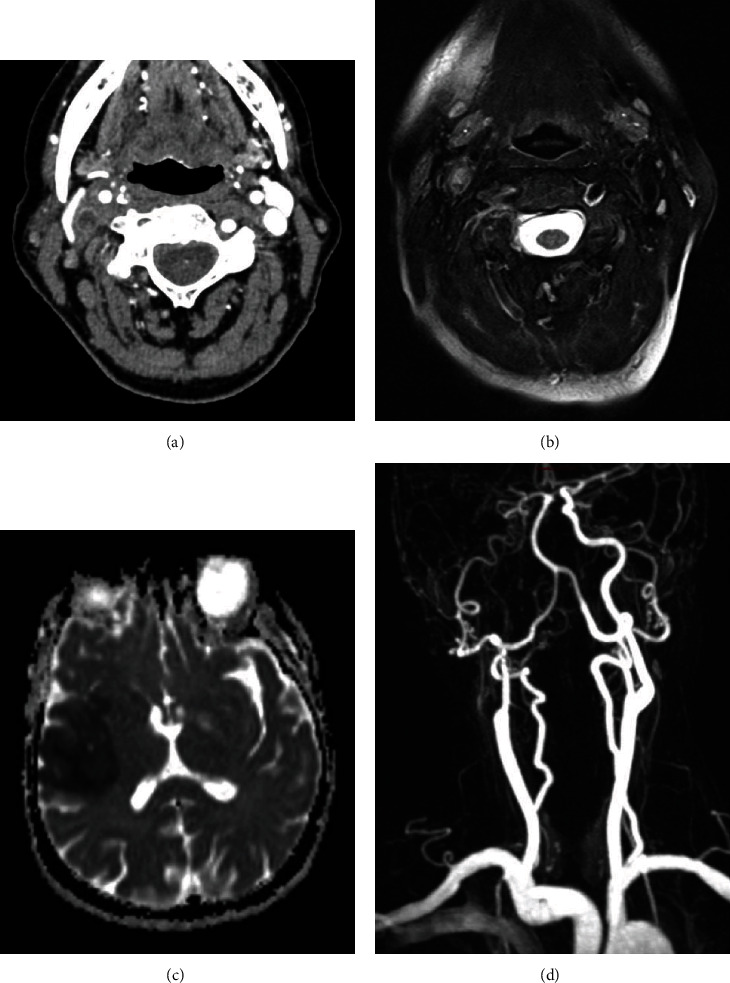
Axial postcontrast CT image (a) demonstrated the presence of vascular occlusion of the right internal carotid artery, immediately above the bifurcation, than confirmed by T2-weighted turbo spin echo (TSE) axial sequence that suggests also the presence of mural inflammation due to edema detection (b). Axial ADC subtraction sequence at the same time demonstrated the presence of a large frontotemporal ischemic lesion (c). Contrast-enhanced MR-angiography after therapy showed a reduction of the segmental occlusion however with persistent filiform contrasting of the right internal carotid artery (d).
